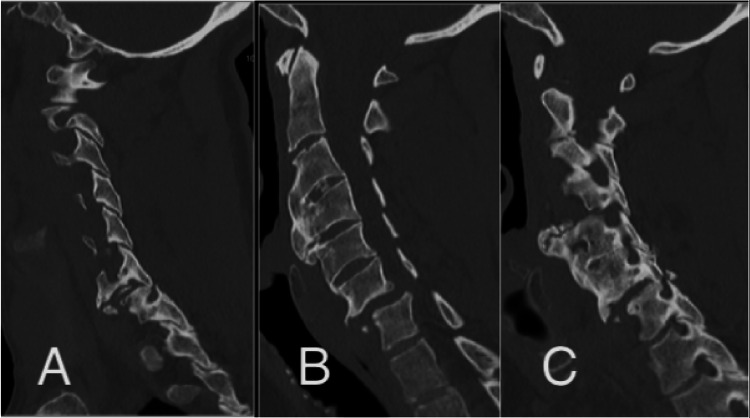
Preoperative CT scan demonstrating an unstable fracture-dislocation at the C6-C7 level, treated with Type 2 MICEPS following ACDF and plating. (A) Left paramedian view; (B) mid-sagittal view; (C) right paramedian view. MICEPS, minimally invasive cervical pedicle screw; ACDF, anterior cervical discectomy and fusion
